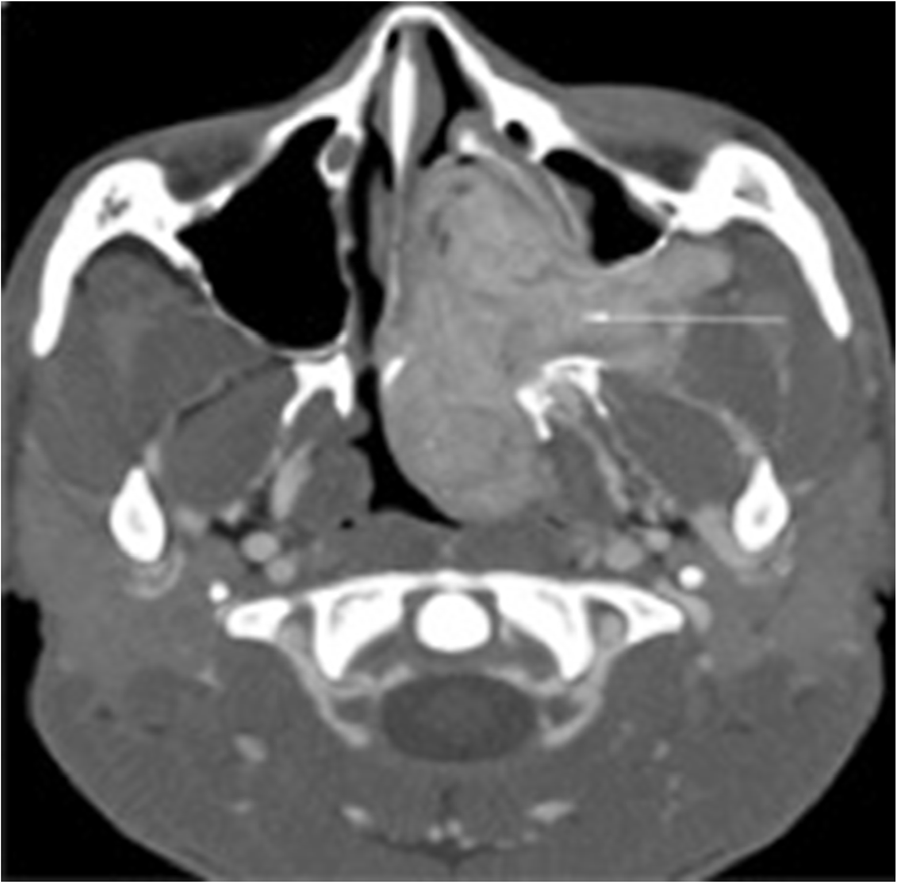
Computed tomography scan showing a Fisch stage 3 JNA
